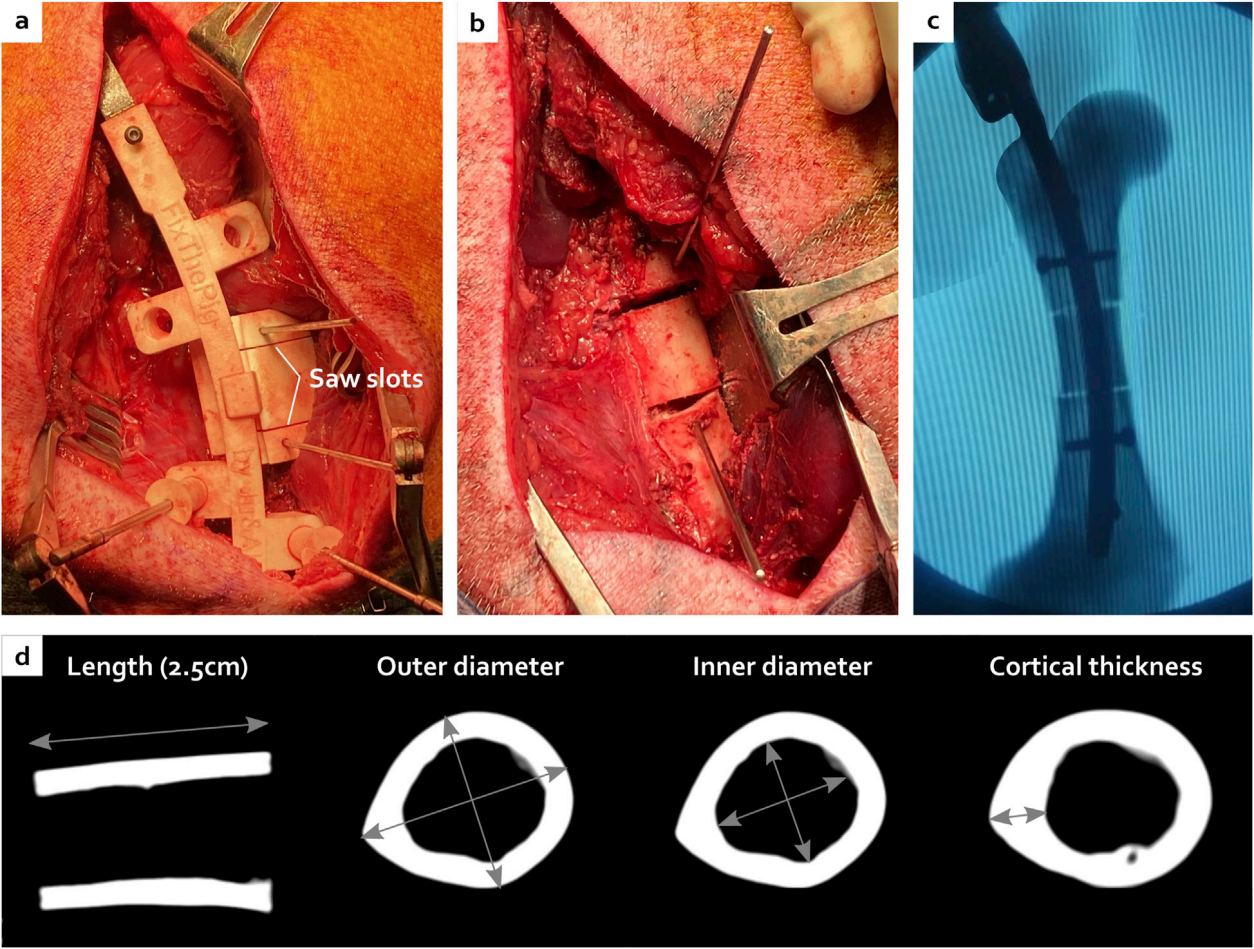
Creation of the critical-size bone defect with the ancillary and the cutting guide (A) leading to a centred ostectomy with reproducible morphology visible during the surgery (B), under fluoroscopy (C) and after CT scan analyses (D).
